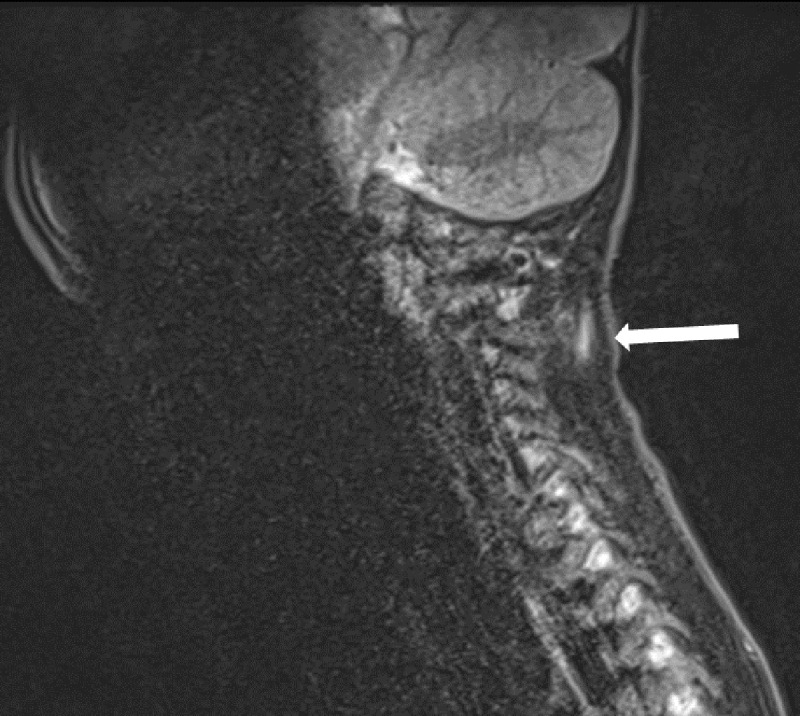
MRI showing left superficial posterior paramedian muscle oedema around C2.
